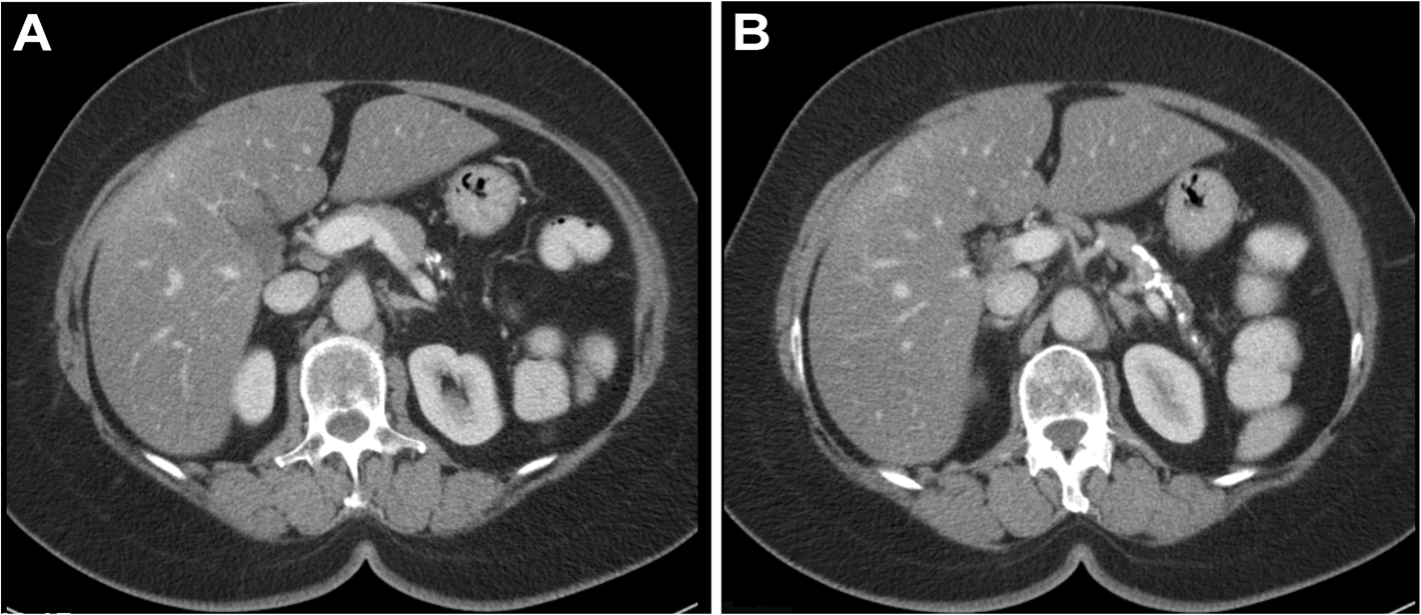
CT scan depicting stones and strictures exclusively located in the tail of the pancreas.
